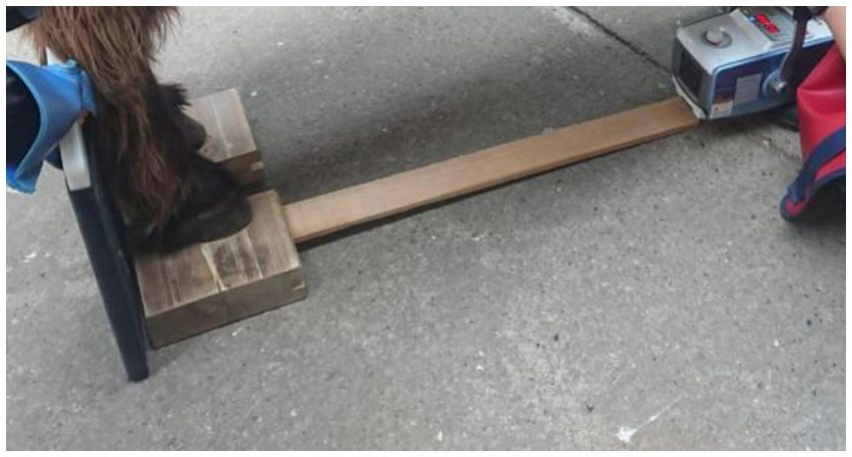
A standardized radiographic technique - the hooves were placed on a specially made wooden block, into which a wooden batten was inserted.
